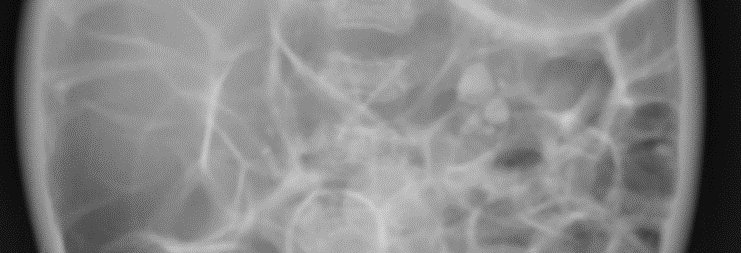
Abdominal X-ray: X-ray represents renal stone in the left kidney.
